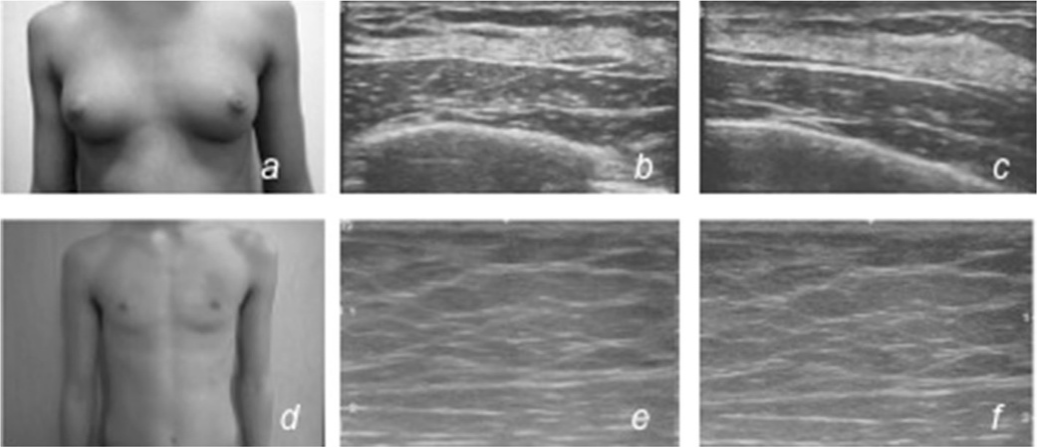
Patient 2. Clinical preoperative breast view (a) and breast ultrasound (b,c) scan showing bilateral gynecomastia. No solid nodular or cystic formations are present on either side; clinical postoperative breast view (d) and breast ultrasound (e,f) scan 1 year after surgery showing a total absence of glandular parenchyma, with muscle planes well represented.
